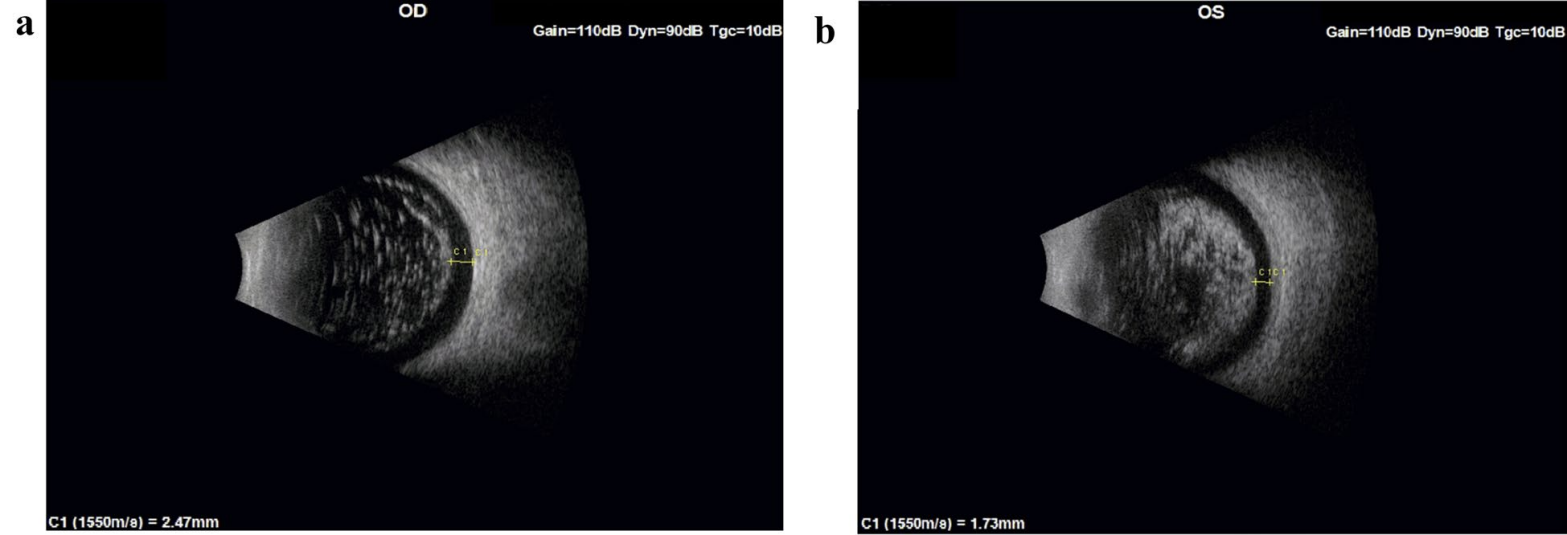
(a) Ultrasound B-mode scan of patient 2 Right Eye. C1: measurement of the closest AH body to distance from the retina. (b) Ultrasound B-mode scan of patient 2 Left Eye. C1: measurement of the closest AH body to distance from the retina
